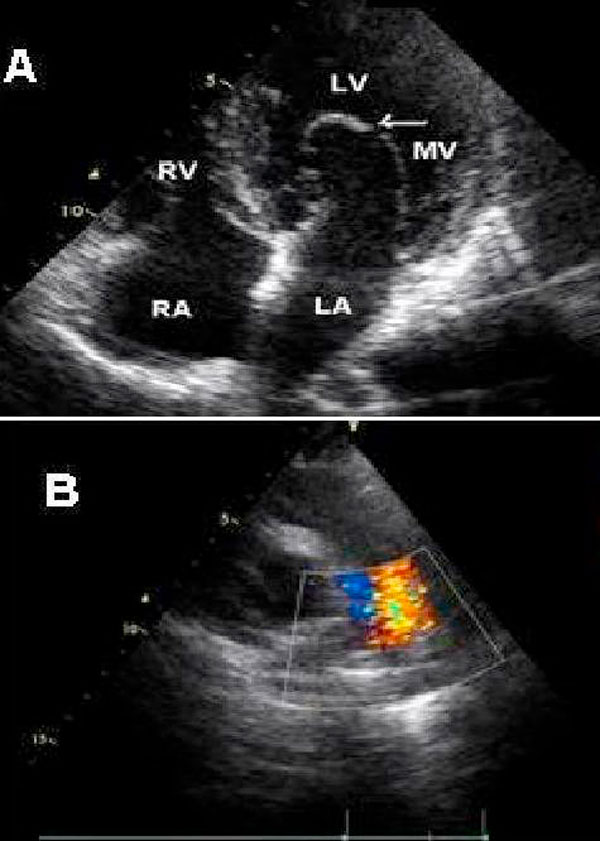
Abnormal mitral valve with congenital elongation and patent ductus arteriosus was shown apical four chamber view (A) and parasternal short axe view (B), respectively.
